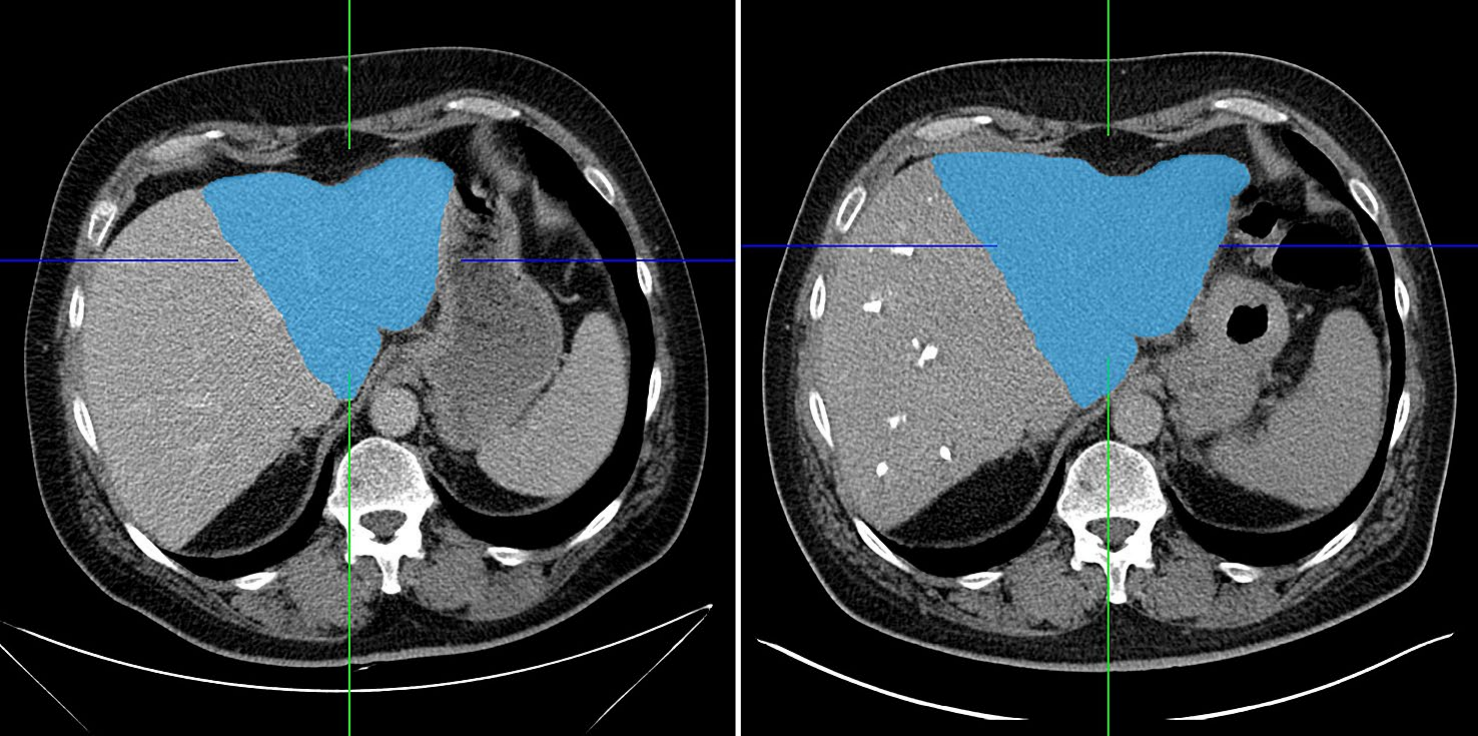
CT scans before (left) and about two months after PVE (right) with volumetry of the FLR (blue)
